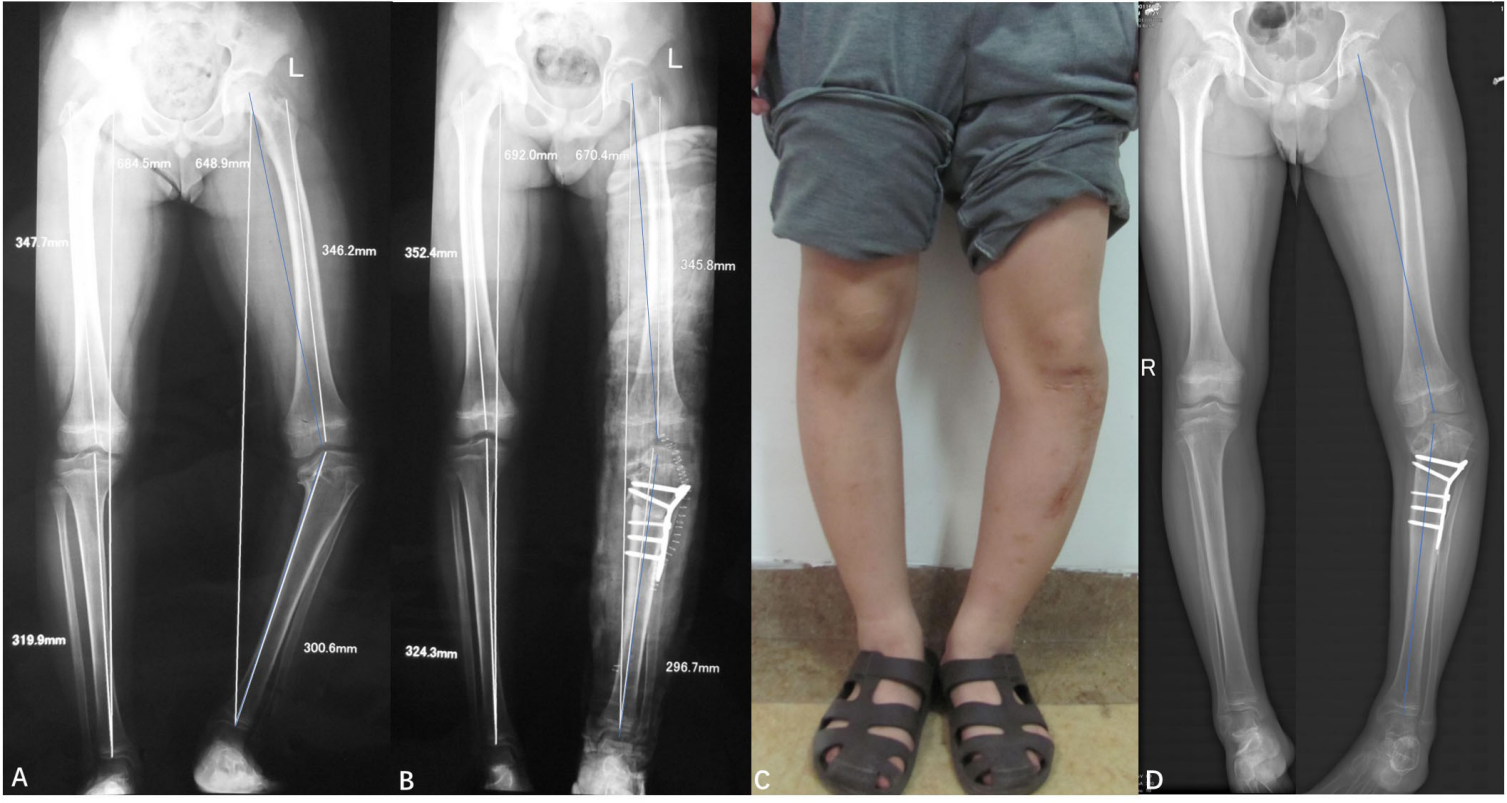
(A) Full-length X-ray of both lower limbs (October 2011, preoperative): Demonstrates varus deformity of the left tibia, wedge-shaped thinning of the medial epiphysis of the proximal tibia, and uneven osteogenesis of the proximal metaphysis. The MAA is 148°. (B) (December 2011, post-secondary surgery): Shows solid internal fixation in an optimal position, MAA: 170°. (C) Photograph of the left lower leg (July 2013, pre-internal fixation removal): Prominent varus deformity with a well-healed 15 cm incision on the anterior aspect of the tibia. (D) Full-length X-ray of both lower limbs (July 2013, pre-internal fixation removal): Reveals good healing of the osteotomy end and stable internal fixation, MAA: 164°.
